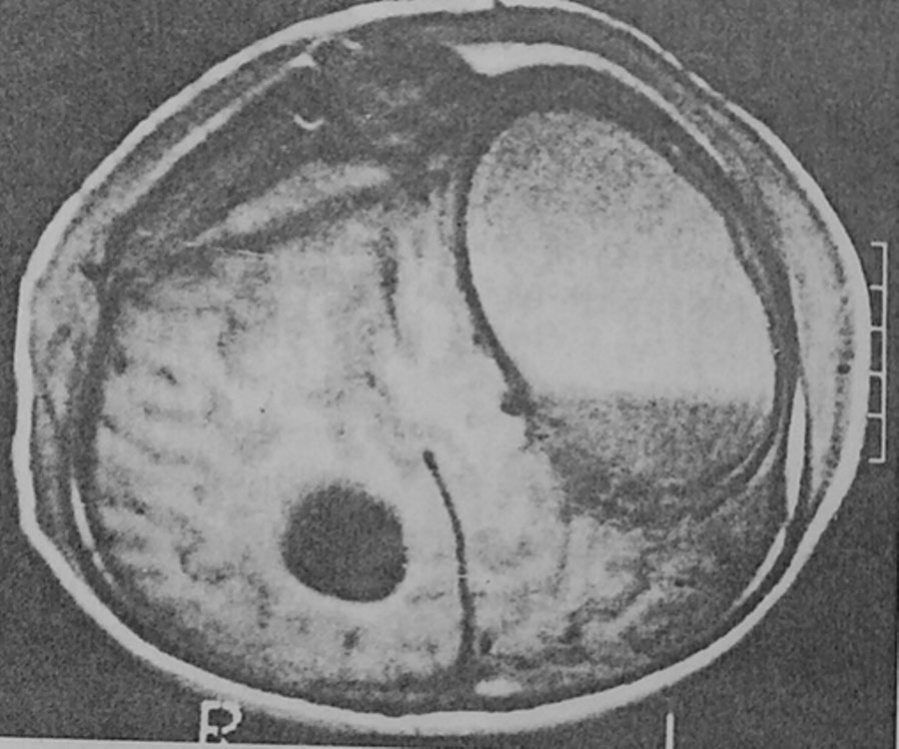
Cranial MRI of the patient prior to the 2nd operation
